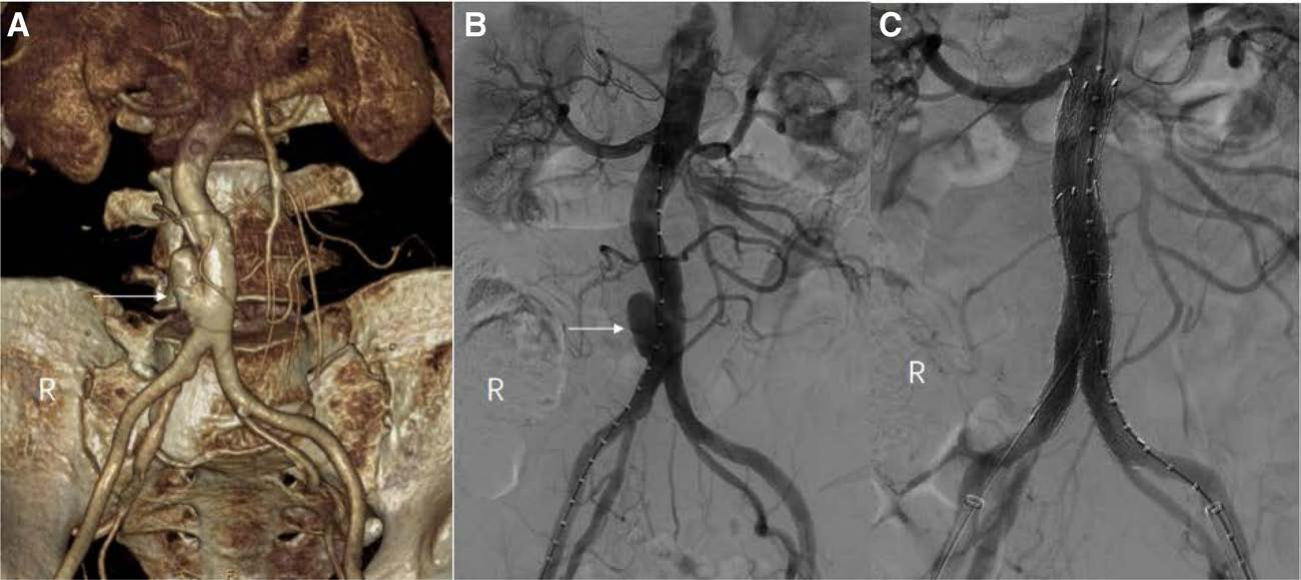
(A) Abdominal aorta CTA shows pseudoaneurysm formation in the lower abdominal aorta. (B) Intraoperative DSA shows a pseudoaneurysm at the end of the abdominal aorta. (C) DSA showed no endoleaks. CTA = computed tomography angiography, DSA = digital subtraction angiography.
